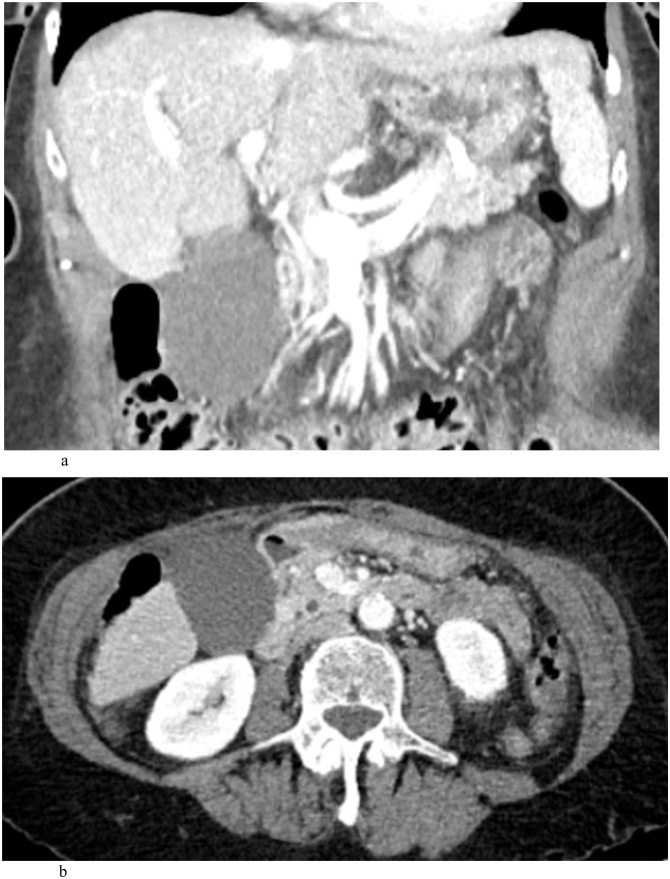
a) Contrast enhanced coronal CT of a gallbladder in a 77-year-old female demonstrating distention and mild pericholecystic fluid, without additional features of cholecystitis. The gallbladder was not enhancing on the scan, and the cystic artery could not be identified. Intraoperatively, she was discovered to have gallbladder volvulus. b) Axial CT of a gallbladder in a 77-year-old female demonstrating distention and mild pericholecystic fluid, without additional features of cholecystitis.
